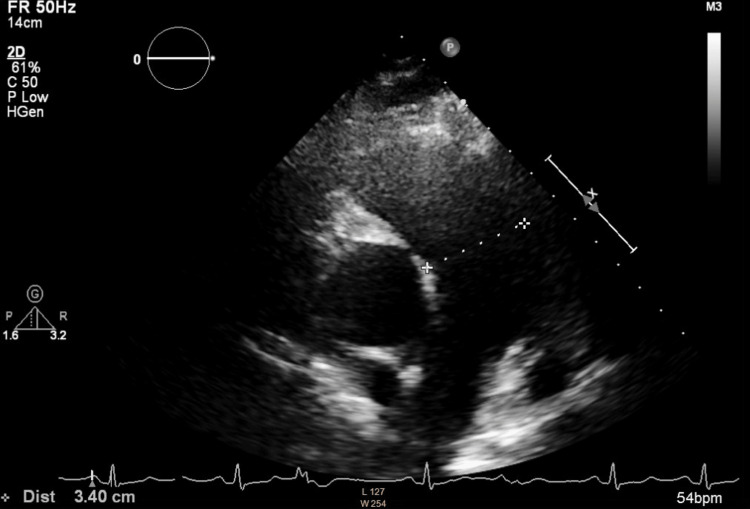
Enlarged pulmonary arteries are seen on the parasternal short-axis view, with the main pulmonary artery measuring 3.4 cm in diameter
